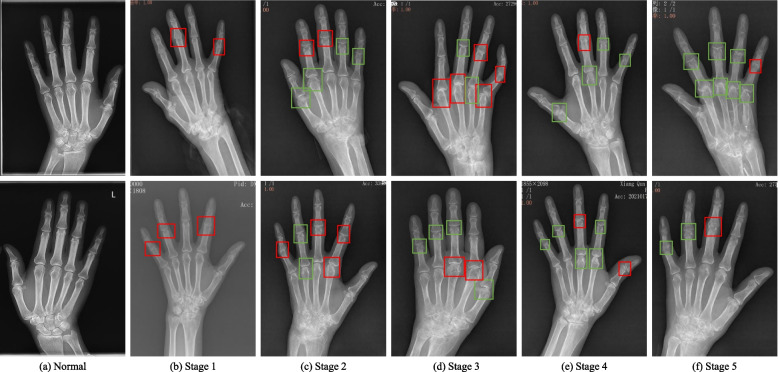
Hand radiographs with RA stage annotations. a-f represent the normal patients and patients with RA at different stages. The top and bottom rows represent the right and left hands, respectively. For the same patient, the red box represents the highest RA stage and the green box represents other stages. For example, for a RA (stage 3) patient, the red box represents stage 3, while the green box represents stage 1 or 2
